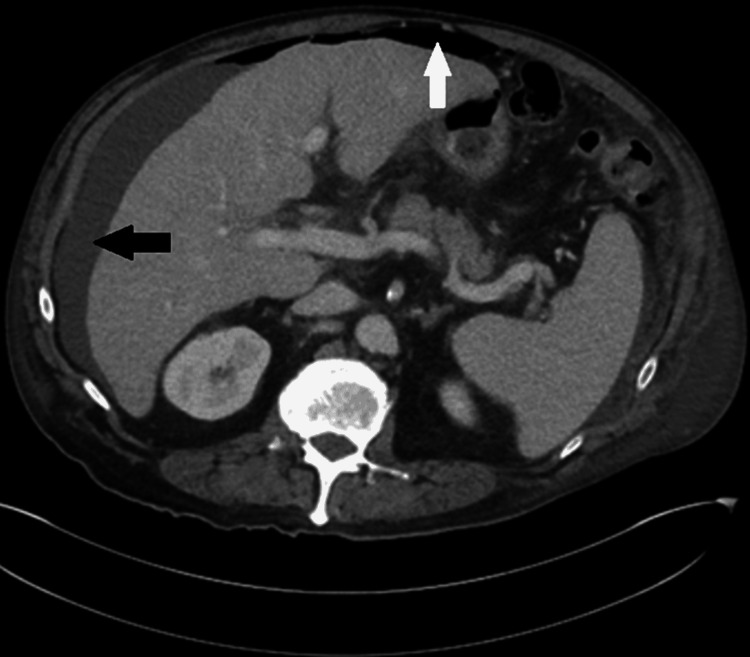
Post-paracentesis abdominal CT showing residual ascites (black arrow) and free air (white arrow), likely post-procedural. The presence of nodular liver contour indicative of cirrhosis, splenomegaly, as well as small gastroesophageal varices.
